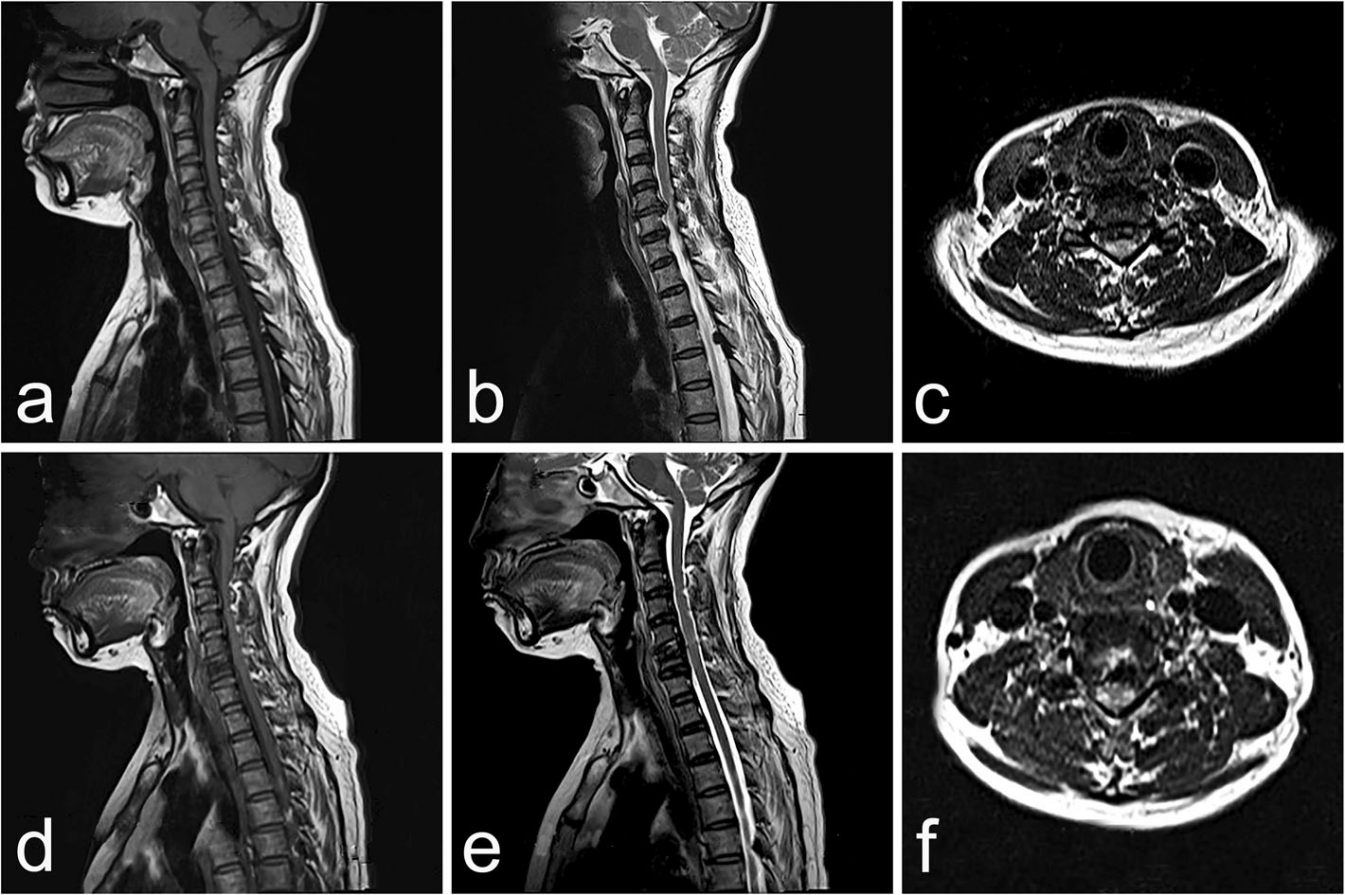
A 43-year-old man was examined due to cervical spondylotic myelopathy. The MRI results showed a broad-based disc hernia at C5/6. The procedure was performed through the channel at C6, and repair was performed with autogenous bone. The postoperative MRI findings showed that the hernia mass was cleared. The preoperative MRI findings, namely, the T1 and T2 weighted sagittal views, are shown (a and b, respectively). Preoperative MRI, T1 weighted axial view (c). Postoperative MRI, T1 and T2 weighted sagittal views (d and e, respectively). Postoperative MRI, T1 weighted axial view (f)
